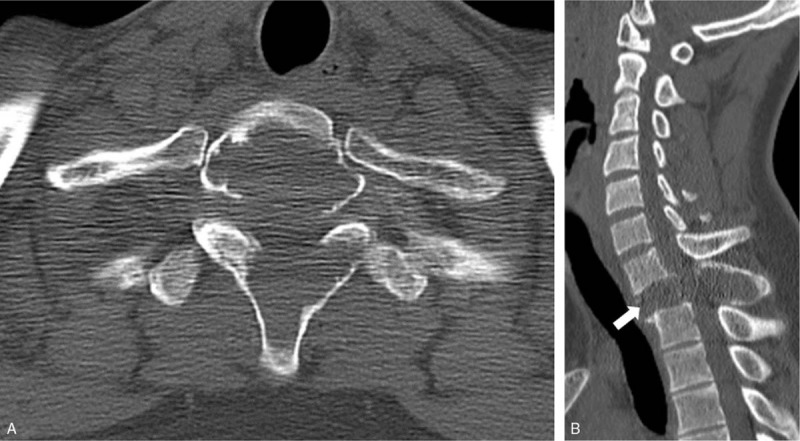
Preoperative computed tomography. (A) and (B) Axial and sagittal image showed osteolytic bony destruction of the T1 body involving the pedicle and spinous process.
